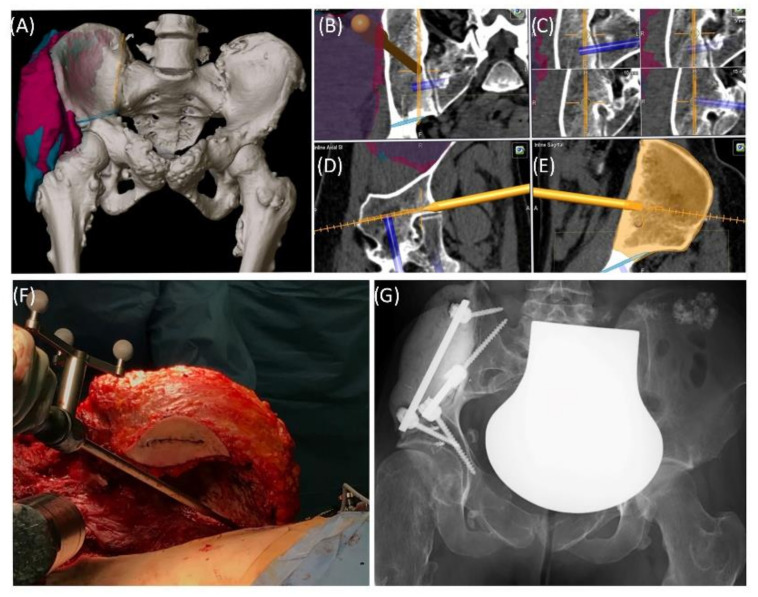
Case 1: Preoperative planning and segmentation of tumor volume components [bony (pink) and soft tissue (teal)] (A); resection planes [transverse (orange) and axial (blue)] during surgery with navigated chisel (orange) (B–E) intraoperative situs during resection (F); postoperative radiograph with reconstructed iliac defect (G).
